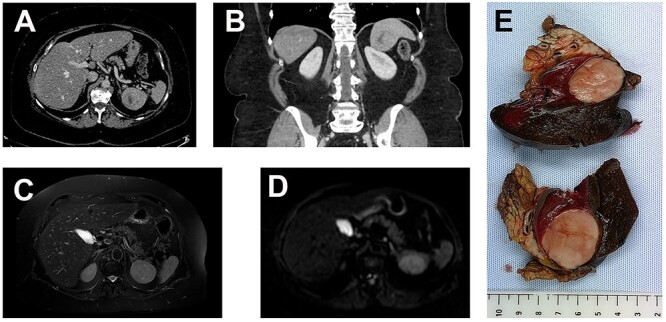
(A, axial) and (B, coronal), portal venous phase CT-scan showing a 43×40×39 mm lesion of the inferior pole of the spleen. The lesion is heterogeneous with a center of necrotic appearance, without calcification and enhancement after injection of the contrast product. A well-defined splenic lesion with high signal intensity on T2-weighted (C) and diffusion-weighted (D) MRI. (E) The macroscopic appearance: tumor is solid, yellow-tan on cut surface with well-circumscribed borders.
